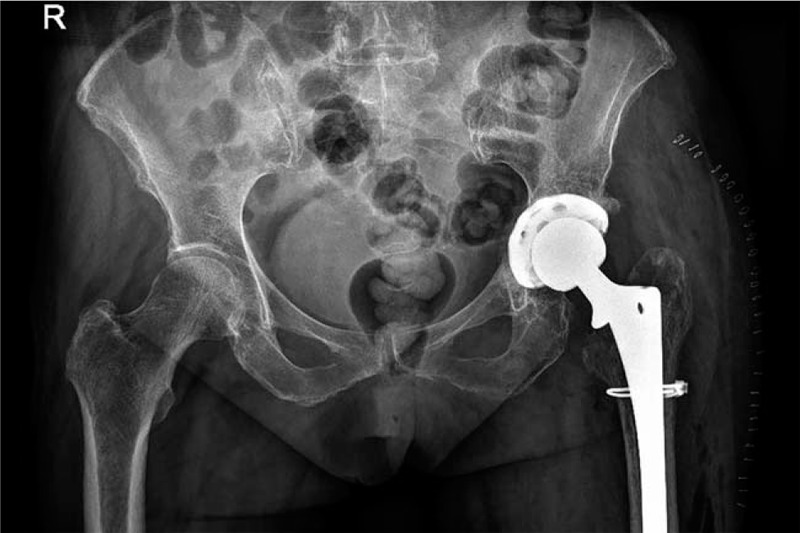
X-ray shows revision after arthroplasty.
